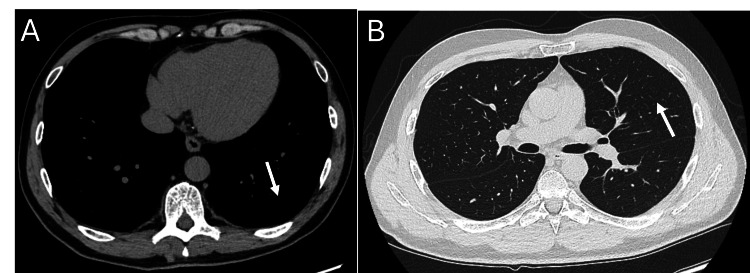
Computed tomography of the chest after three-month treatment. (A) Computed tomography of the chest shows complete resolution of the left-sided pleural effusion (arrow). (B) The lung window shows complete re-expansion of the retro-obstructive atelectasis in the lingula (arrow) and clearance of endobronchial mucus impaction within the lingular bronchi.
